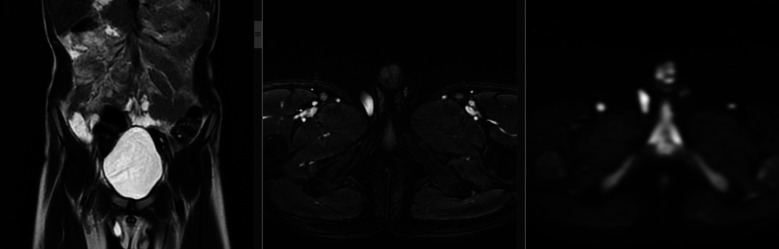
7-year-old boy presenting with right inguinal testis on MRI, appears of high signal intensity. On (a) coronal T2W, (b) axial T2W fat - sat, and (c) axial DWI.
